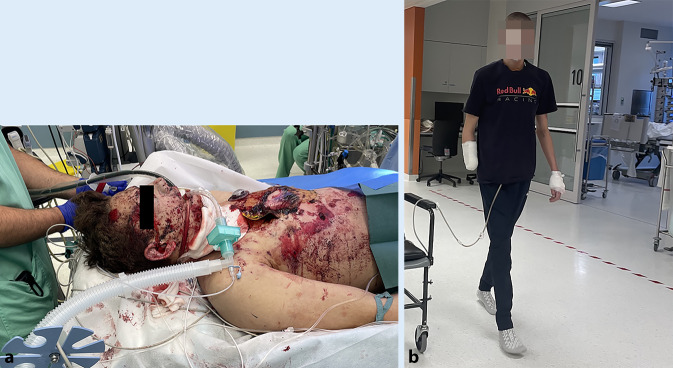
Am ersten postoperativen Tag wird eine CT-Kontrolle durchgeführt. Erfreulicherweise finden sich keine Zeichen eines hypoxischen Hirnschadens, der maximale NSE-Wert ergibt 27 µg/l (Normwert < 12,5 µg/l). Es folgen tägliche transthorakale echokardiographische Untersuchungen, welche die Dichtigkeit des eingenähten Patch und eine langsame Verbesserung der kardialen Auswurfleistung zeigen. Beatmung und Oxygenierung funktionieren problemlos.
